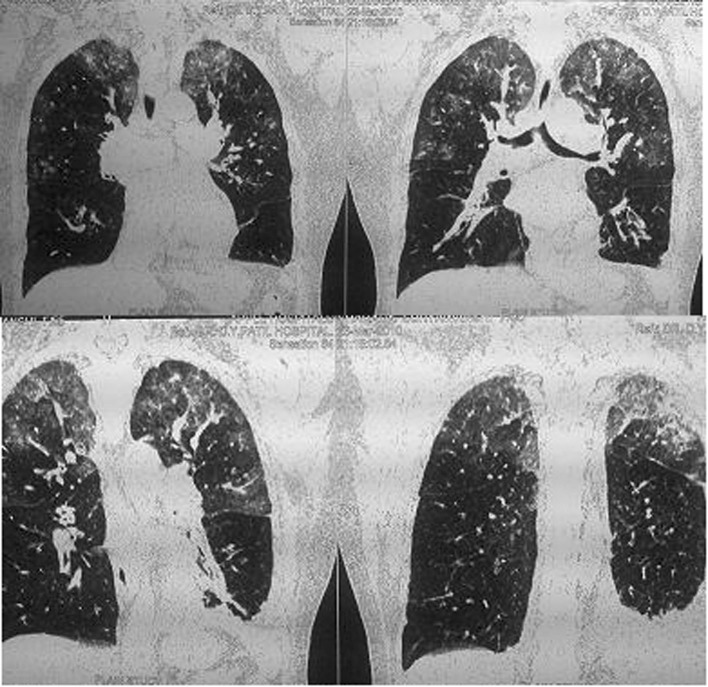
HRCT chest (23.03.2010) showing bilateral diffuse patchy infiltrates.
